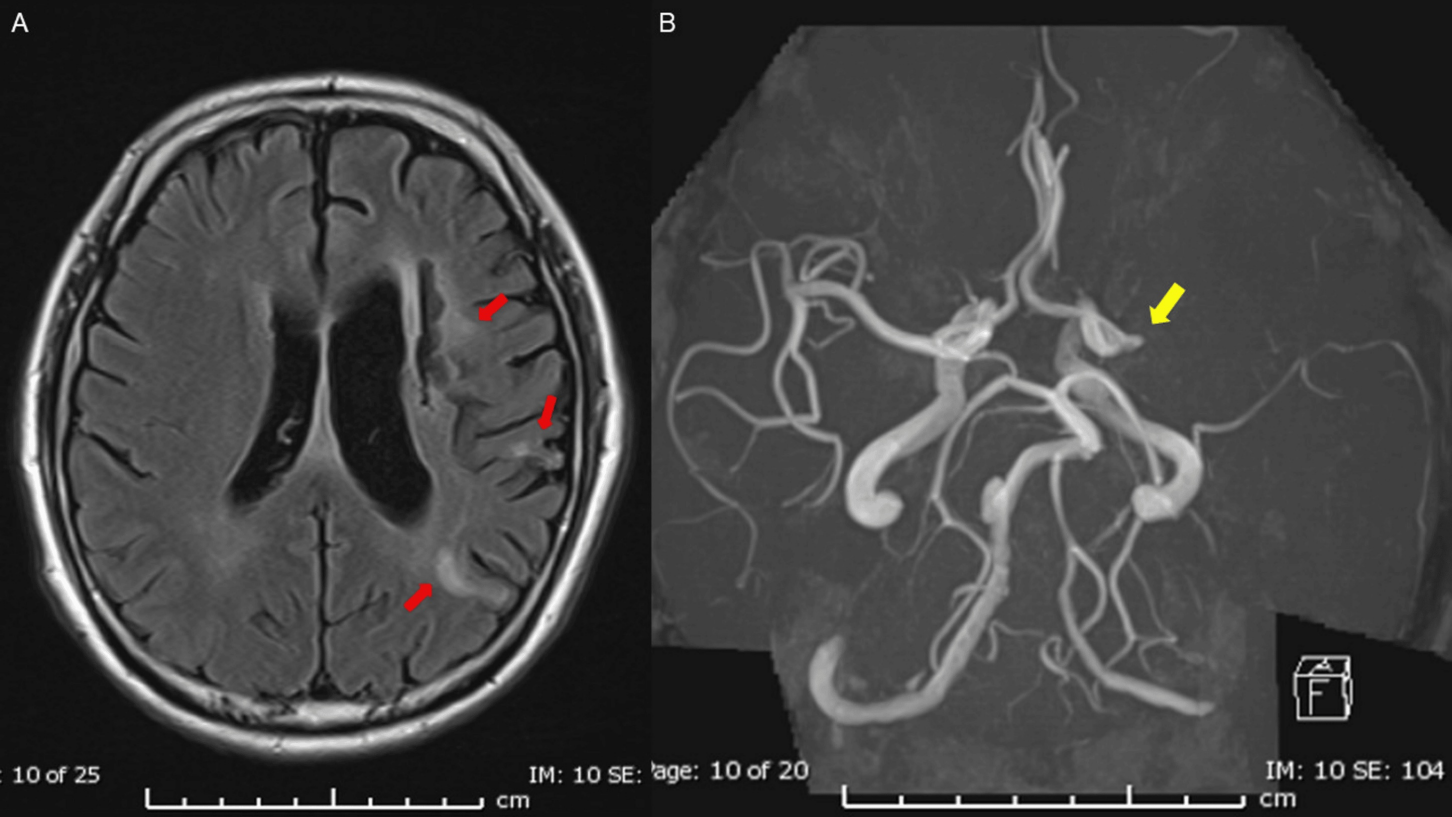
Preoperative head magnetic resonance imaging (MRI) (A) MRI showed an old cerebral infarction in the left cerebrum (red arrows). (B) MR angiography showed occlusion of the left middle cerebral artery (yellow arrow).
